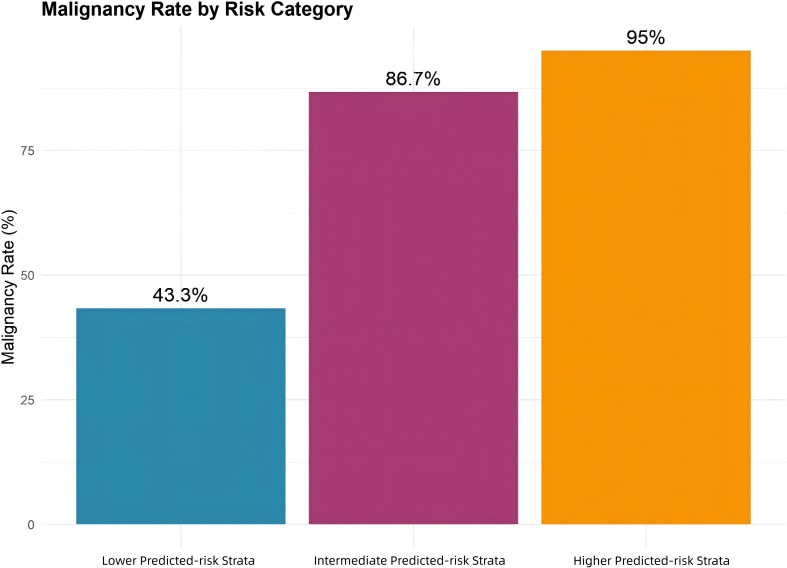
Clinico–quantitative imaging for preoperative prediction of malignancy in pulmonary nodules ≤ 3 cm. Predictors include sex, symptoms at detection, time from detection to surgery, nodule radiographic appearance, consolidation-to-tumor, suspicious radiologic features, nodule size, min CT attenuation (per 100 HU), and max CT attenuation (per 100 HU). To estimate an individual’s risk, locate the patient’s value on each predictor scale, draw a vertical line to the Points axis to assign points, sum the points on the Total Points axis, and project downward to obtain the Predicted Probability of Malignancy. Higher total points indicate a higher risk of malignancy. (model derived from multivariate logistic regression; internal performance is reported in Figures 1-3).
